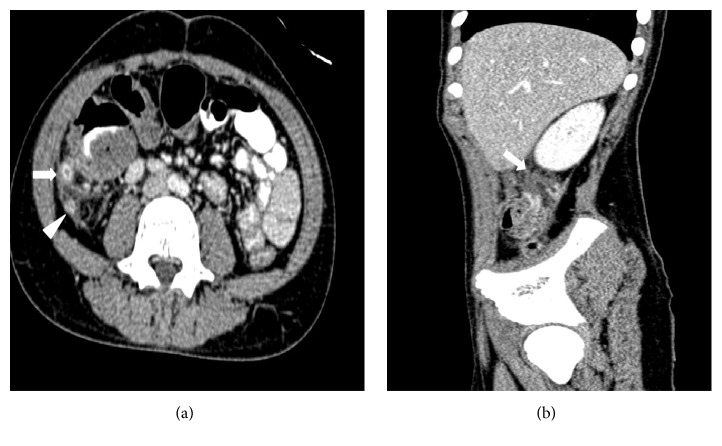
Contrast enhanced CT showing long, inflamed, and retrocaecal appendix with a distal kink. (a) Axial image showing inflamed (enhancing) appendix (proximal part-arrow and tip-arrow head). (b) Sagittal image showing the nonenhancing mid portion of the appendicular wall with kink (arrow) and no periappendicular collection.
